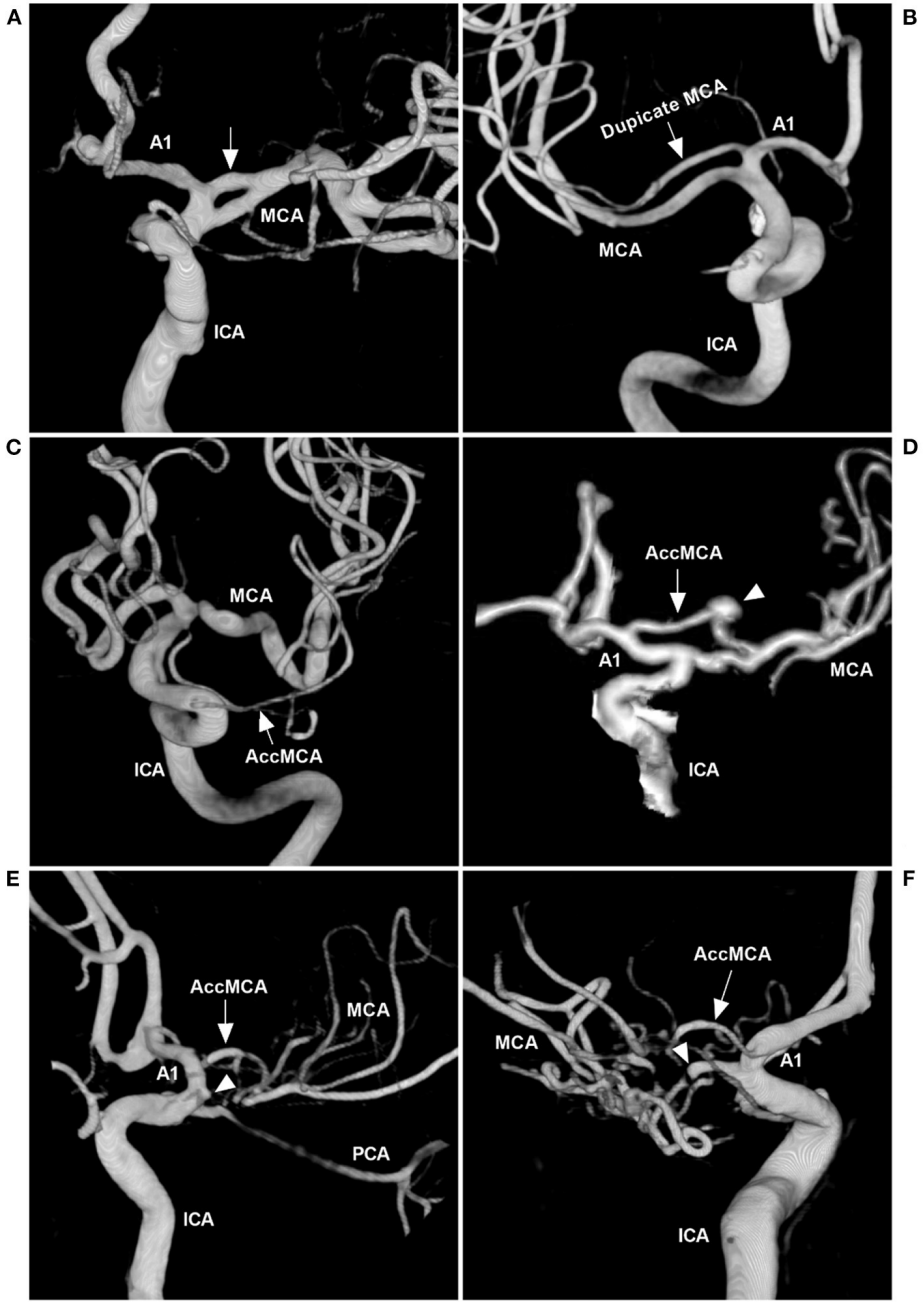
MCA anomalies on angiography. (A) Three-dimensional DSA of the ICA showing a fenestration (arrow) at the beginning of the MCA. (B) Three-dimensional DSA showing a duplicate MCA (arrow). (C) Three-dimensional DSA showing a type 1 AccMCA (arrow) from the ICA trunk. (D) Three-dimensional DSA showing a type 2 AccMCA (arrow) with complete anastomosis between the A1 middle segment and MCA forming a large fenestration; the arrowhead indicates an aneurysm. (E) Three-dimensional DSA showing a twig-like MCA supplied by the AccMCA (arrow) from the A1 end; the MCA did not supply the twig-like MCA, and the MCA origin presented with a protrusion (arrowhead). (F) Three-dimensional DSA showing that the twig-like MCA was supplied by the AccMCA from the A1 origin (arrow); the MCA supplied the twig-like MCA (arrowhead). A1, first segment; AccMCA, accessory middle cerebral artery; CT, computed tomography; DSA, digital subtraction angiography; ICA, internal carotid artery; MCA, middle cerebral artery; PCA, posterior cerebral artery.
